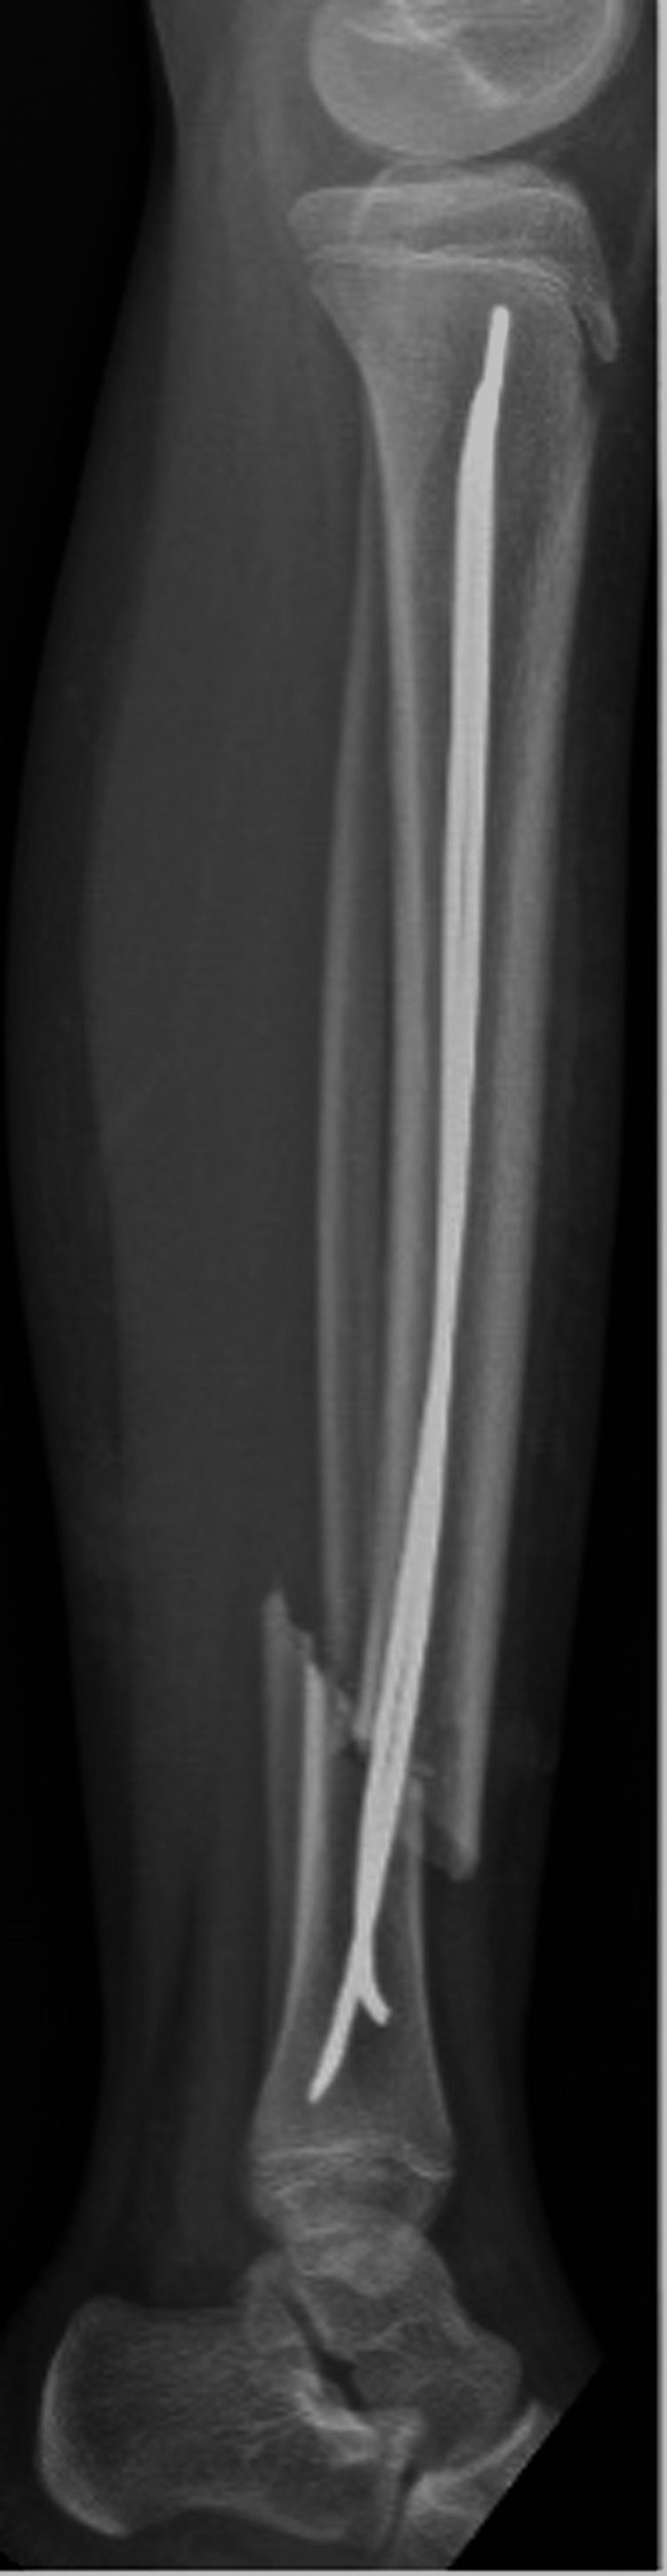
One-month post-operative lateral tibia-fibula radiographs of flexible nailing of the tibia.
